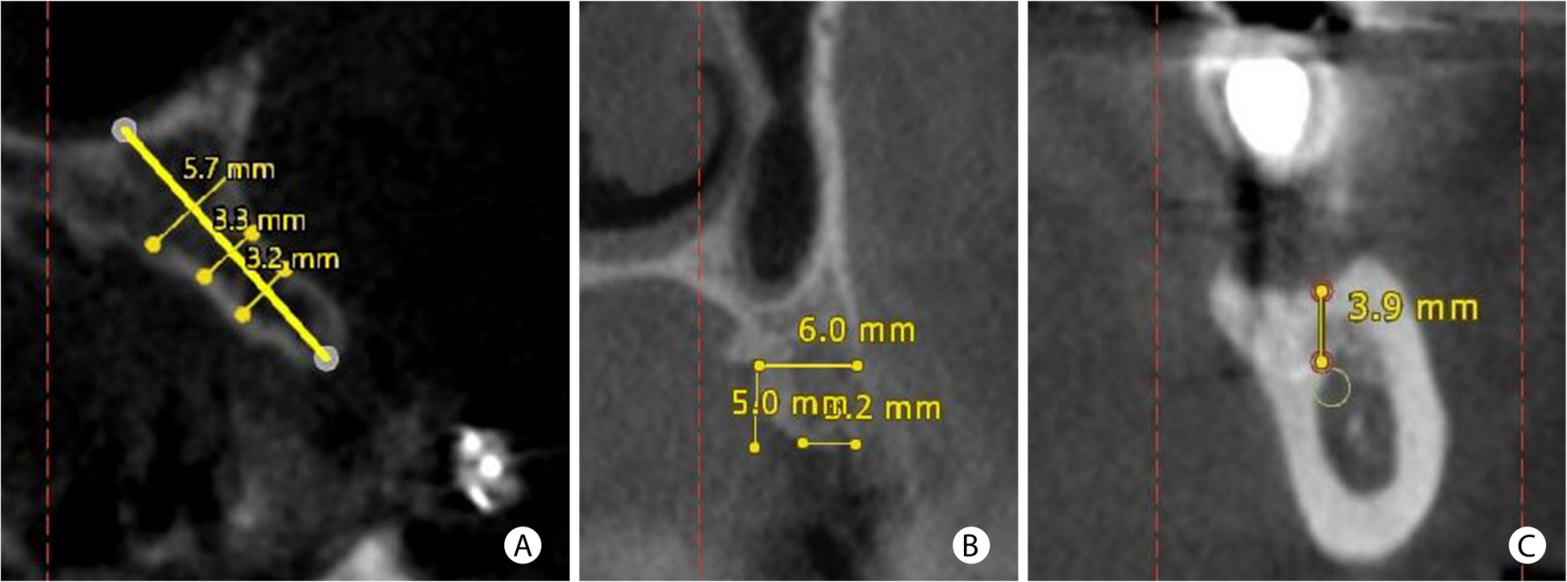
The methodology used to measure bone defects in the CBCT. a For horizontal bone defects, the tomographic measurement was performed at three different points, and three horizontal lines recorded bone thickness at 5, 7, and 11 mm from the alveolar crest. b In regions close to the maxillary sinus with a horizontal deficiency in the remaining bone crest, the measurement was performed 5 mm from the bone crest. c Vertical bone defect measured from the viable edge of the alveolar crest to the lower alveolar nerve
